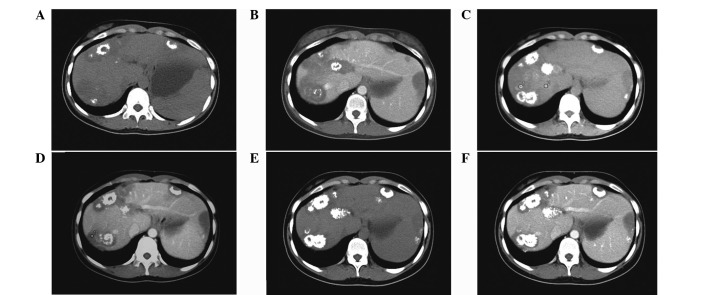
Three-year follow up CT images of one patient (no. 4) who did not receive any specific therapy. (A and B) First plain CT image showed multiple hypoattenuating coalescent nodules in the peripheral region of the liver with capsular retraction and mild calcification. (C and D) Second CT (one-year later) showed no change in tumor size, but an increase in calcification. (E and F) Third CT examination (two-years later) reconfirmed the regression of the tumor with the augmentation of calcification replacing the previous tumor cells. CT, computed tomogrphy.
